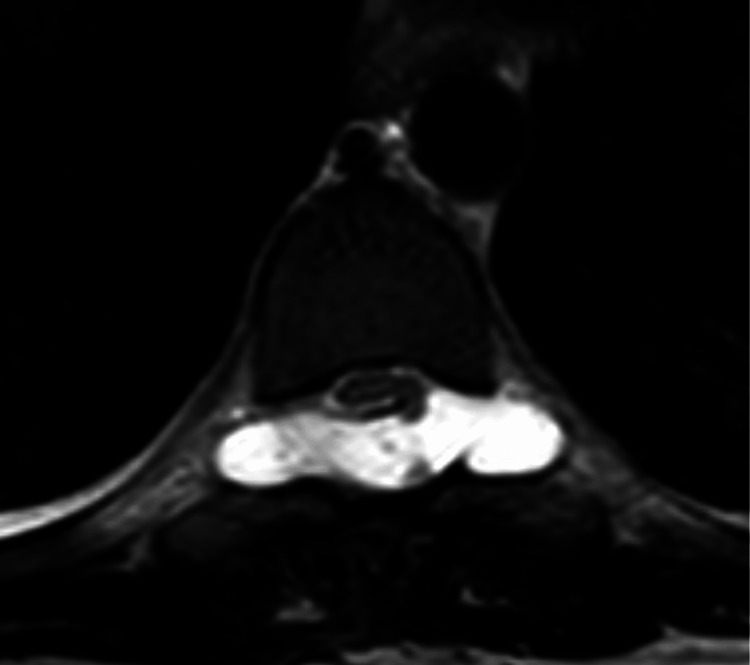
Axial T2-weighted MRI at the T8 level demonstrating a posterior left dural defect with focal spinal cord herniation.
